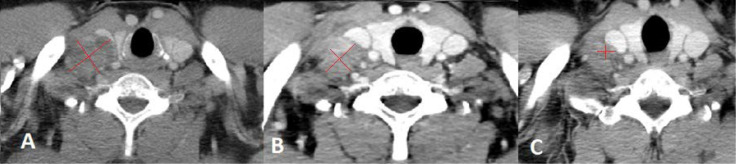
Chest CT scan performed at baseline (A), after the first cycle of Pembrolizumab (B) and after the fourth cycle of therapy (C). Image A shows the enlarged supraclavicular lymphnode 2.7 cm x 3.8 cm, compressing the right internal jugular vein. Image B shows the right supraclavicular lymphadenopathy moderately reduced in size 2.5 cm x 3.2 cm, with less evident signs of compression on the internal jugular vein. Image C shows a further dimensional reduction of the right supraclavicular lymphnode 1.04 cm x 1.15 cm, which is more than halved with respect to baseline
